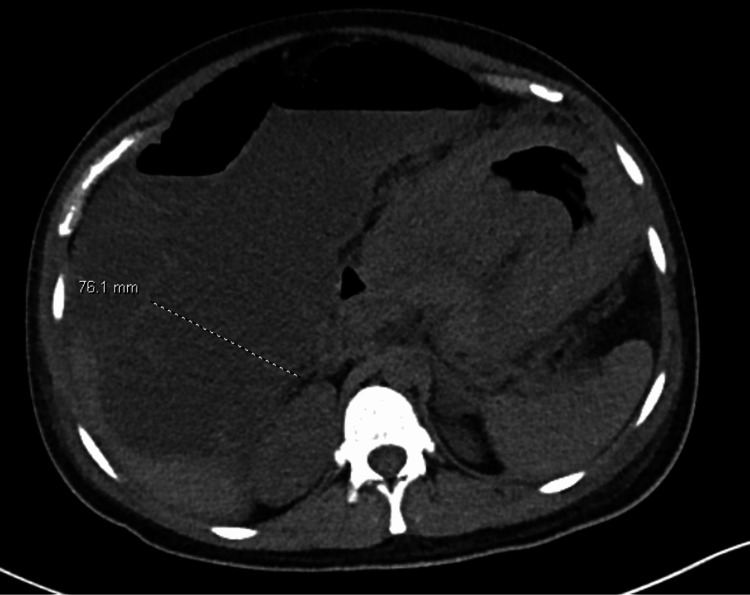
Coronal views of CT abdomen/pelvis showing ascending colon dilated to 7.6 cm diameter
